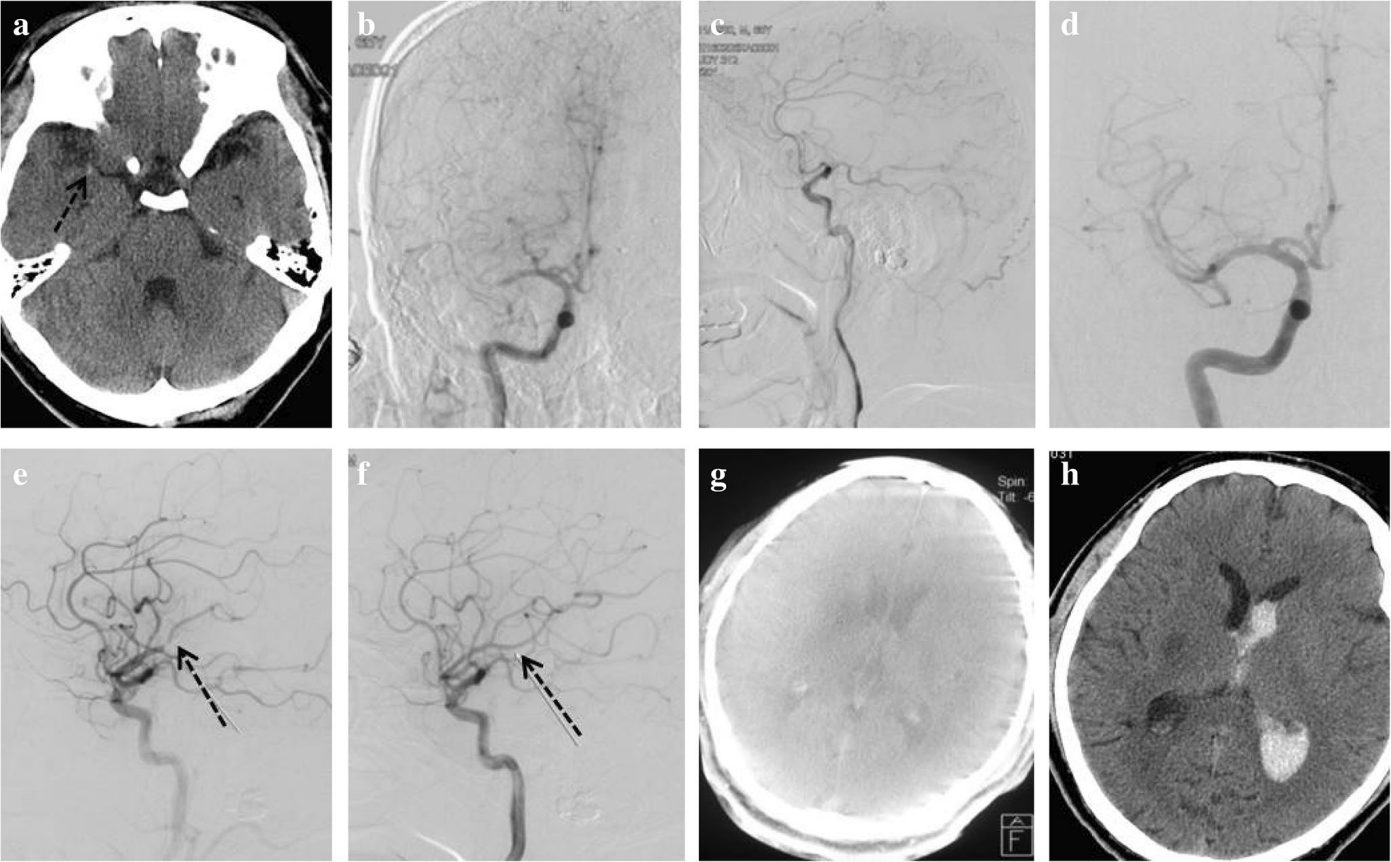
Images of case 1. a Non-contrast CT: a hyperdense right MCA (black arrow). b Cerebral angiography: occlusion of the M1 segment of right MCA from frontal image. c Cerebral angiography: occlusion of the M1 segment of right MCA from lateral image. d Cerebral angiography: spontaneous recanalization of the M1 segment of right MCA before endovascular intervention. e Cerebral angiography: thrombus moving to MCA bifurcation 5 min later (black arrow). f Cerebral angiography: thrombus disappeared (black arrow) and complete recanalization of the right MCA. g Dyna-CT image: no hemorrhagic transformation. h Follow-up non-contrast CT at 24 h after IVT: right thalamus hemorrhage
